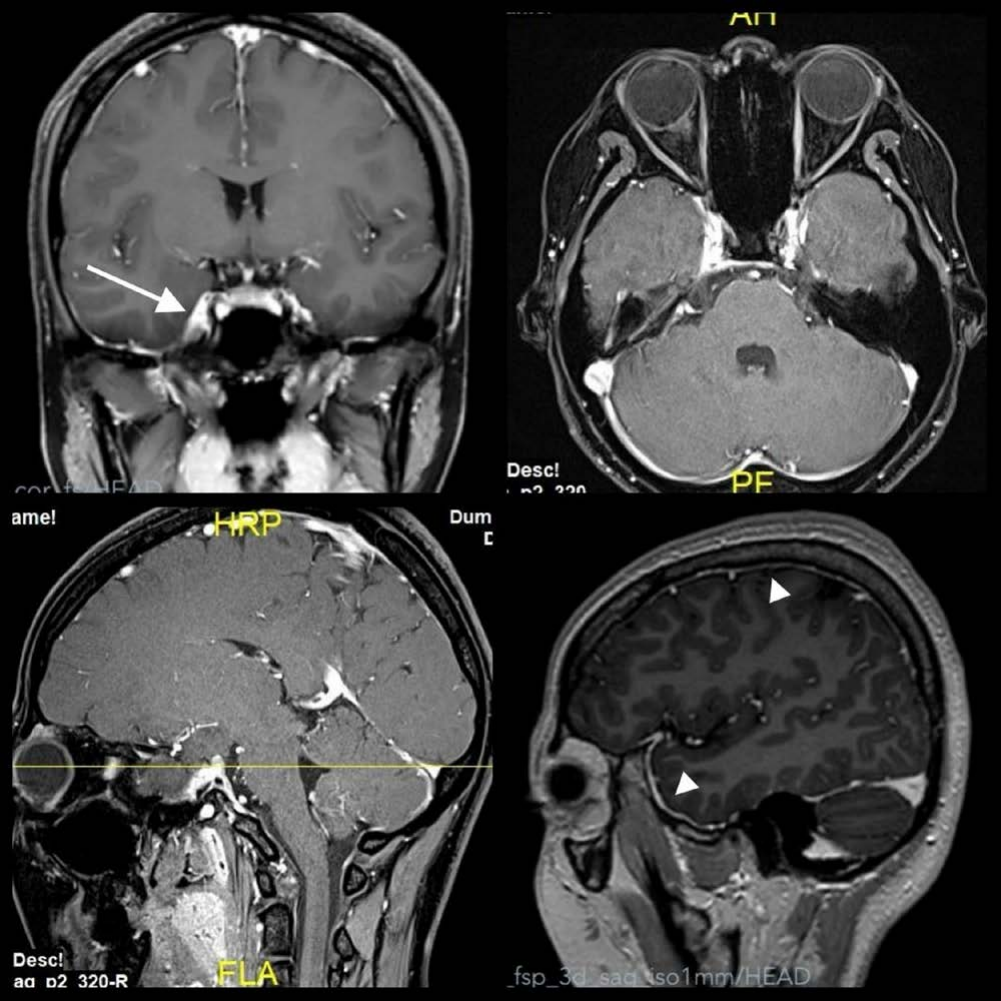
MRI revealed gadolinium enhancement of the thickened prefrontal cortex dura mater (arrowheads) and clivus region near the cavernous sinus on the right side (long arrow). MRI = magnetic resonance imaging.
